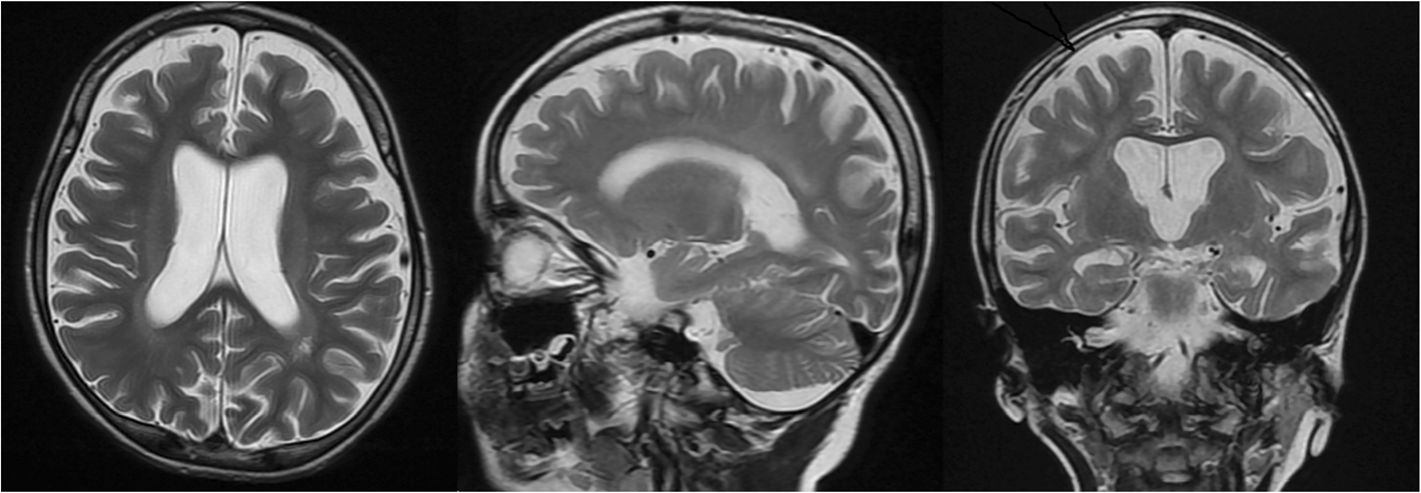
Brain magnetic resonance imaging of patient
